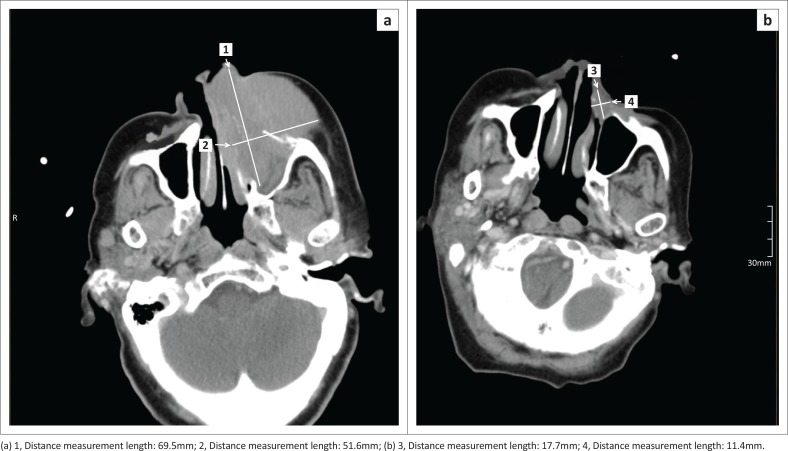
Plasmablastic lymphoma presenting as a maxillary mass. Initial computed tomography (CT) (a) and restaging CT (b), demonstrating a partial response.
